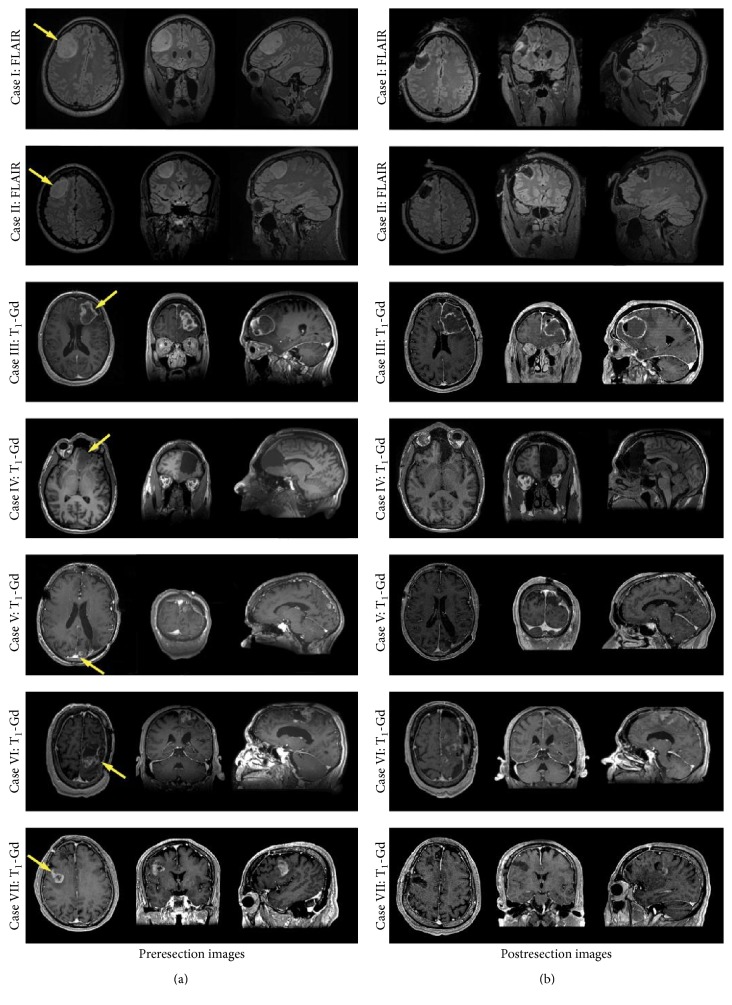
((a) and (b)) Pre- and postresection MR images (representative axial, coronal, and sagittal views); different MR imaging sequences have been included to highlight the various tumours. Three of the patients (Cases I, II, and IV) had nonenhancing glioma WHO grade III and four patients (Cases III and V–VII) enhancing lesions following administration of gadolinium contrast agent. Postresection MR images show the extent of resection for each patient. Some residual enhancement is evident in patients I, VI, and VII.
